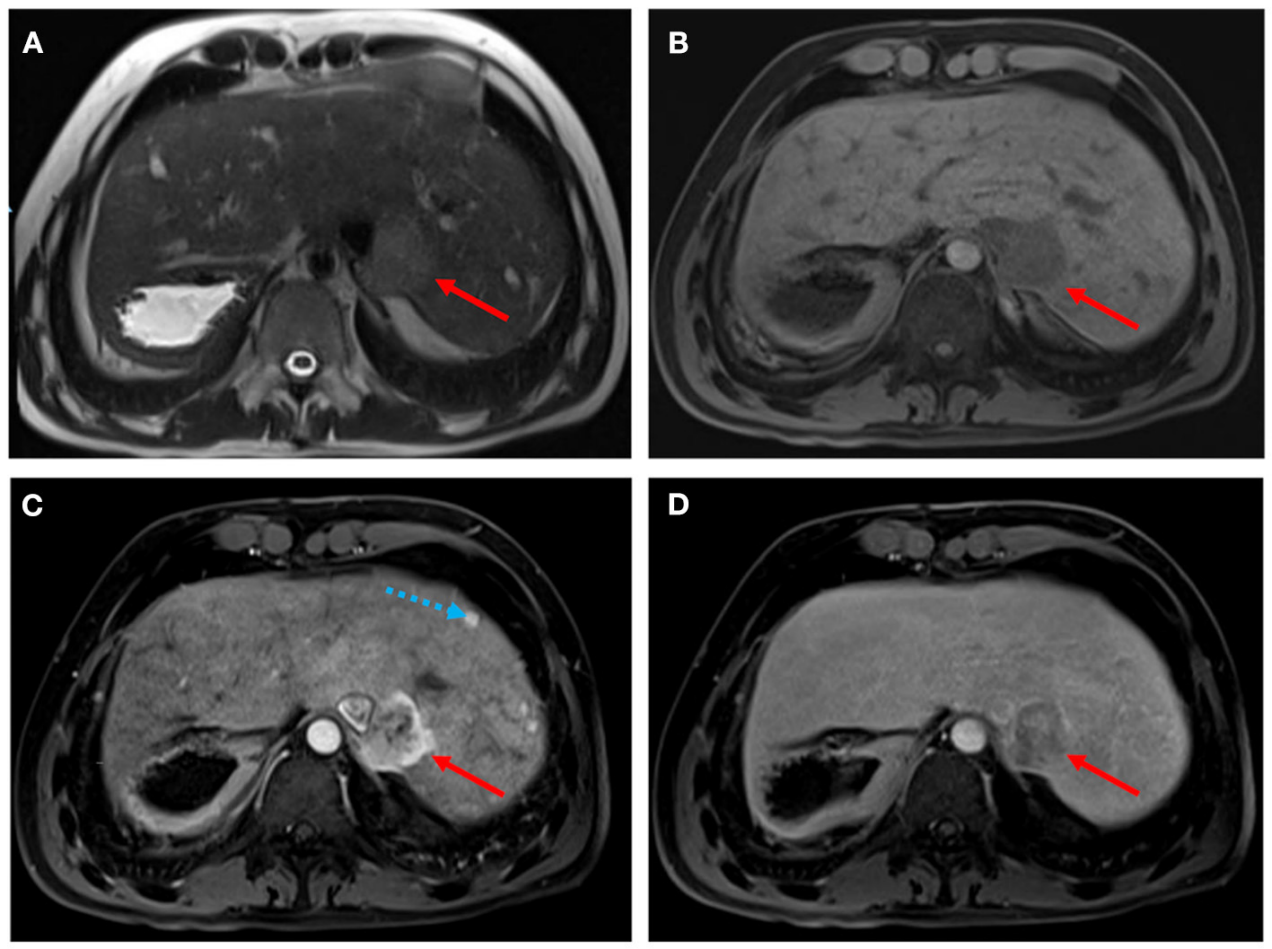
Hepatocellular carcinoma in a 30-year-old man with Fontan palliation. Axial T2- and T1-weighted MR images (A,B) show a 4 cm paracaval solid lesion with smooth margins and posterior hepatic capsule bulge (arrows). The tumor shows heterogeneous hyperintensity in the arterial phase of the dynamic contrast-enhanced sequence (C), and typical washout in the delayed phase (D). The background liver is diffusely heterogeneous after contrast injection, with multiple small arterial-enhancing foci [dotted blue arrow in (C)] without delayed washout, in keeping with hypervascular regenerative hepatic nodules.
